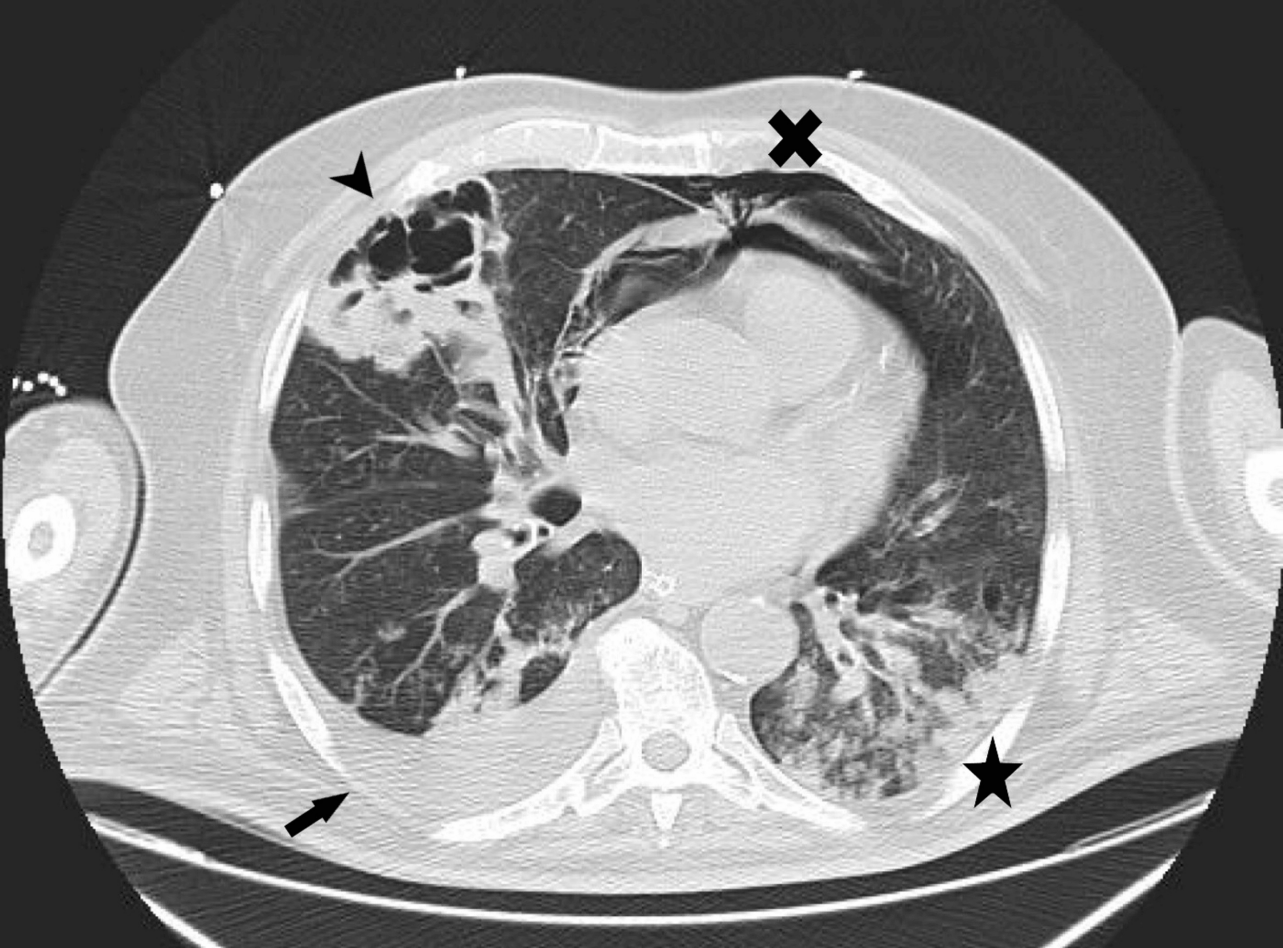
Axial CT scan of the patient's thorax. In the image, a cavitary lesion with thick, heterogeneous walls, suggestive of necrotizing pneumonia, is indicated by the arrowhead. The arrow points to a right-sided pleural effusion with passive atelectasis of the adjacent lung. Just below the cross, a pneumomediastinum is identified and the star indicates parenchymal infiltrates with areas of consolidation.
